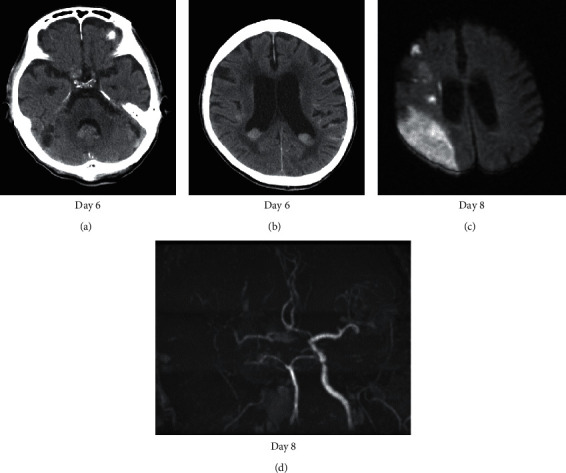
Day 6: head CT showing a subarachnoid hemorrhage (a), (b). Day 8: brain MRI showing a right middle cerebral artery infarction on DWI (c) and MRI showing right internal carotid artery occlusion (d).
